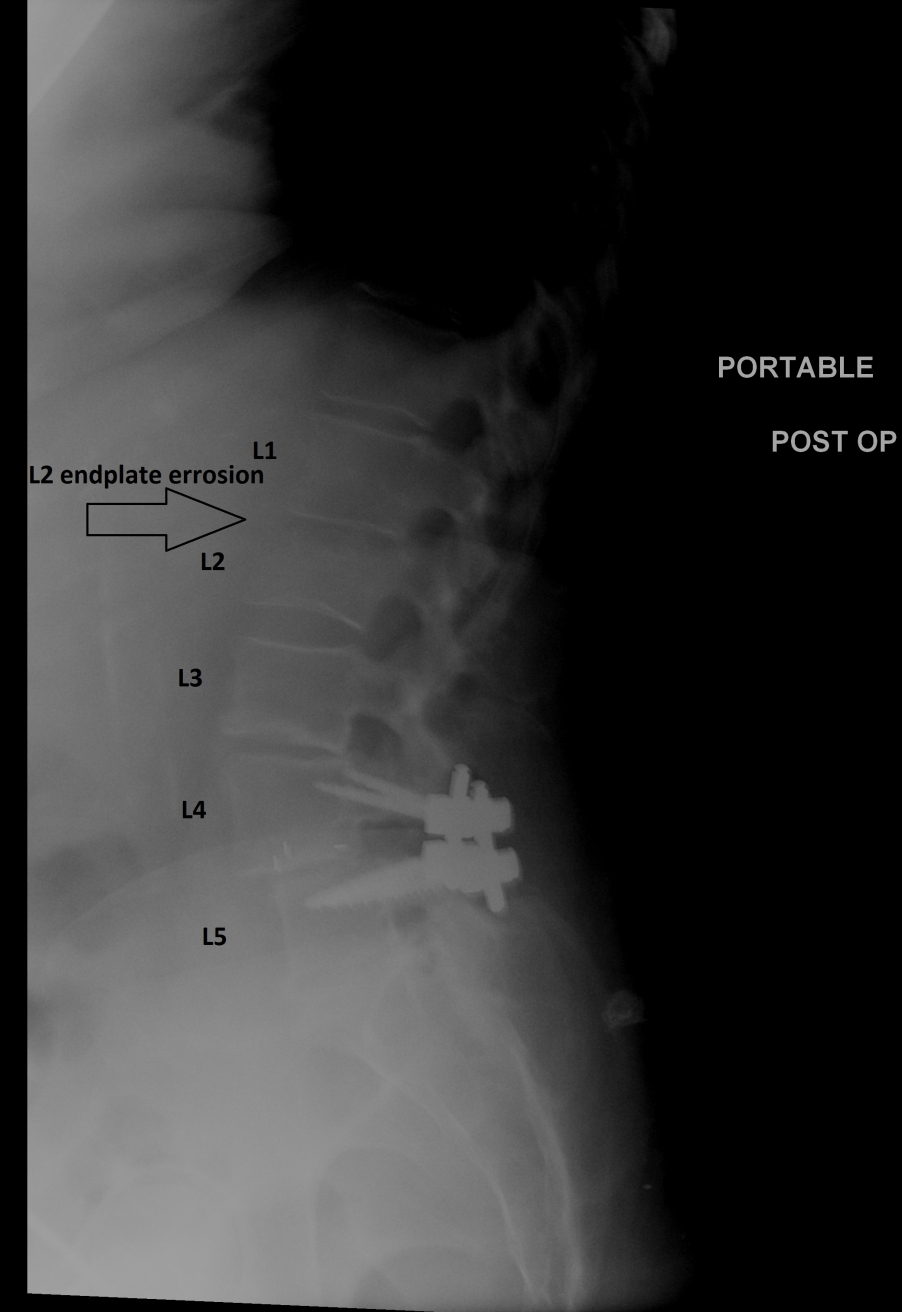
Postoperative X-rays with L2 Erosive Changes
